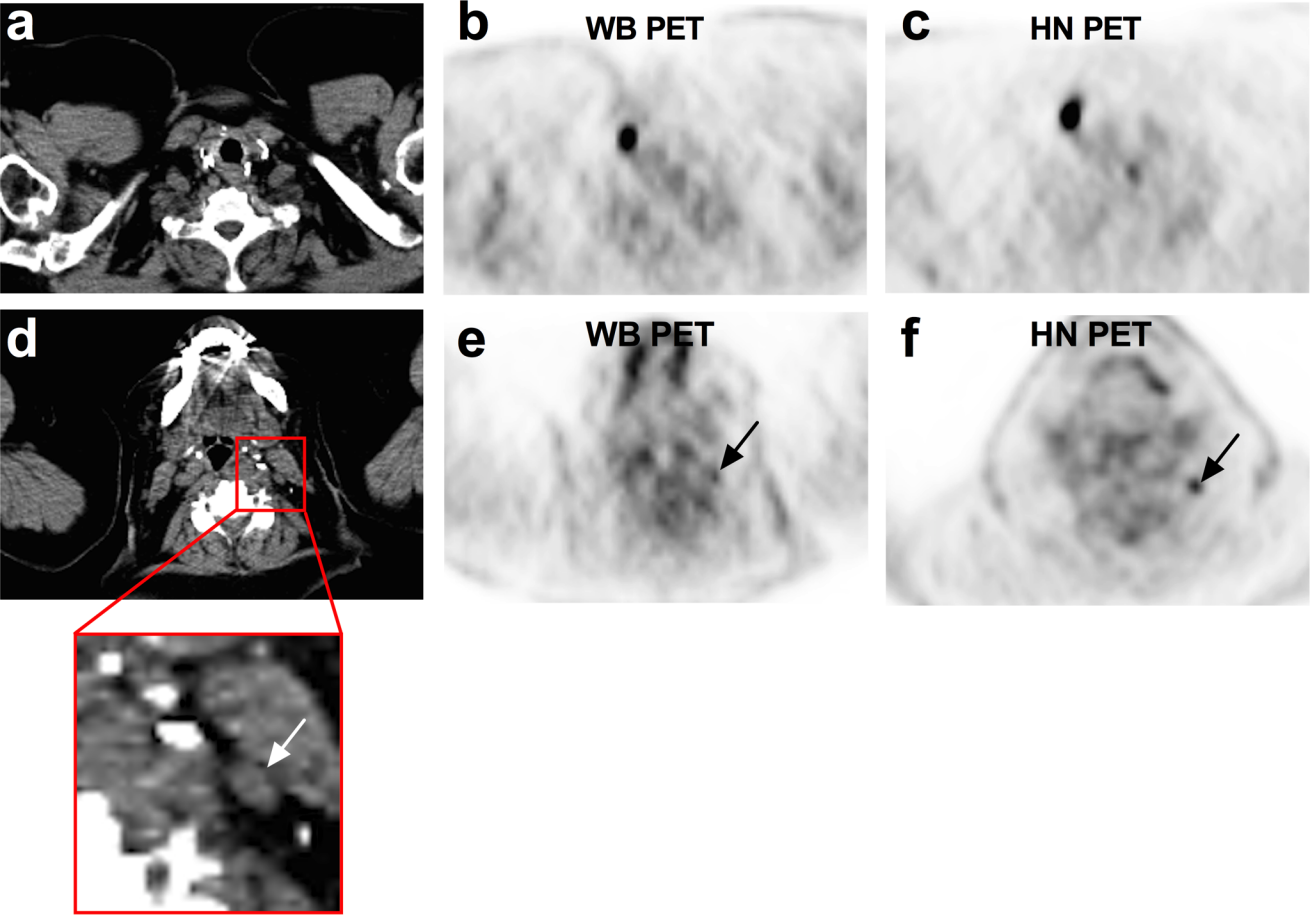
PET/CT study in a 74-yr-old patient (Patient 11) with a pT4aN1bM1 papillary thyroid cancer (tall-cell variant). She presented with a detectable serum Tg level under levothyroxine (14 ng/ml) 5 years after initial diagnosis. Both whole-body (WB) and head and neck (HN) PET (Panels b and c) evidenced a grade 5 FDG focus in front of an abnormal LN (short-axis: 10 mm; long axis: 16 mm) in the right lateral compartment IV (Panel a). A small grade 4 FDG focus was also reported by both readers on HN PET (Panel f) in front of an apparently normal LN (short-axis: 4 mm; long axis: 8 mm) located in the left lateral compartment II (Panel d). This FDG focus was not reported on WB PET (Panel e). Node/muscle background ratio was 6.0 on HN PET vs. 3.9 on WB PET. After bilateral neck dissection, pathology confirmed HN PET/CT data.
